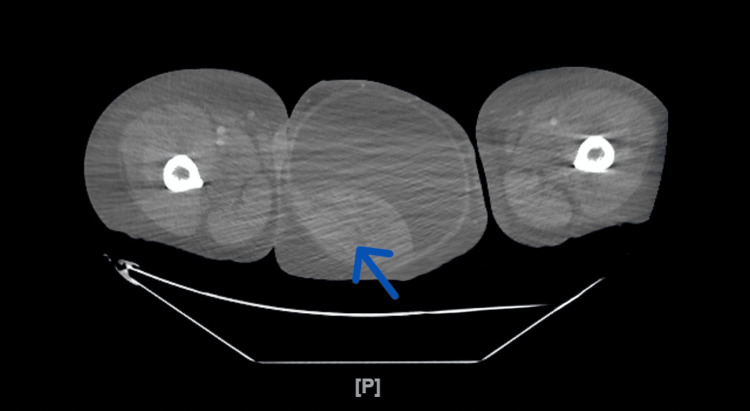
CT of the abdomen and pelvis with contrast showing left testicular heterogenous mass (blue arrow)
